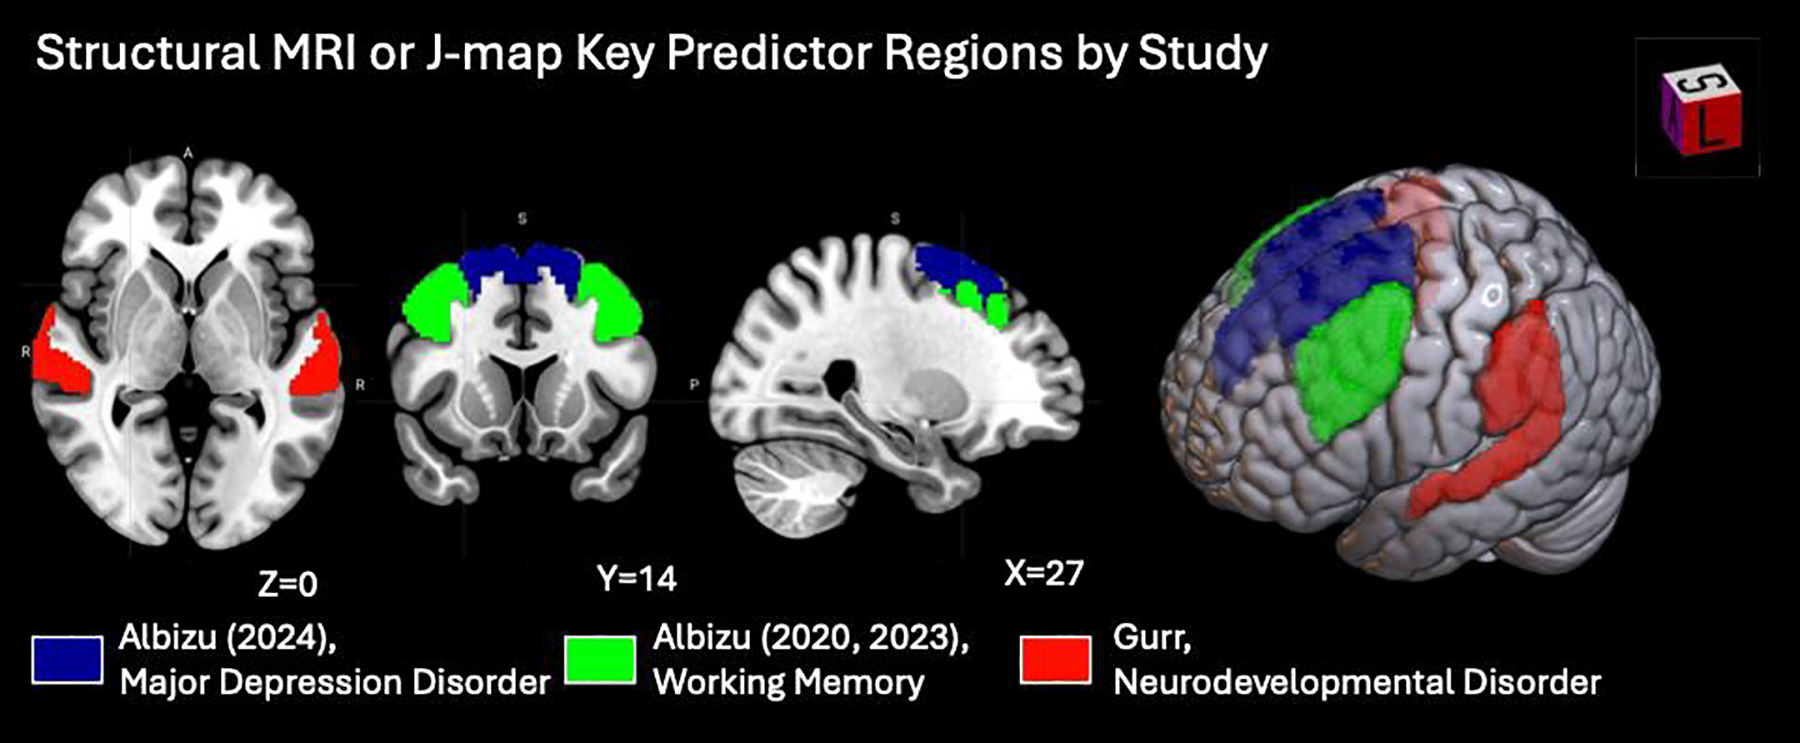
Structural MRI or J-map predictor regions across ML studies of tDCS and related therapeutic indications. Visualizations were generated using MRIcroGL v26.0.1 (https://www.nitrc.org/), with overlays mapped onto the MNI152 template supplied by the software. Albizu (2020, 2023) refers to two separate studies conducted by Albizu et al., published in 2020 and 2023, respectively.
